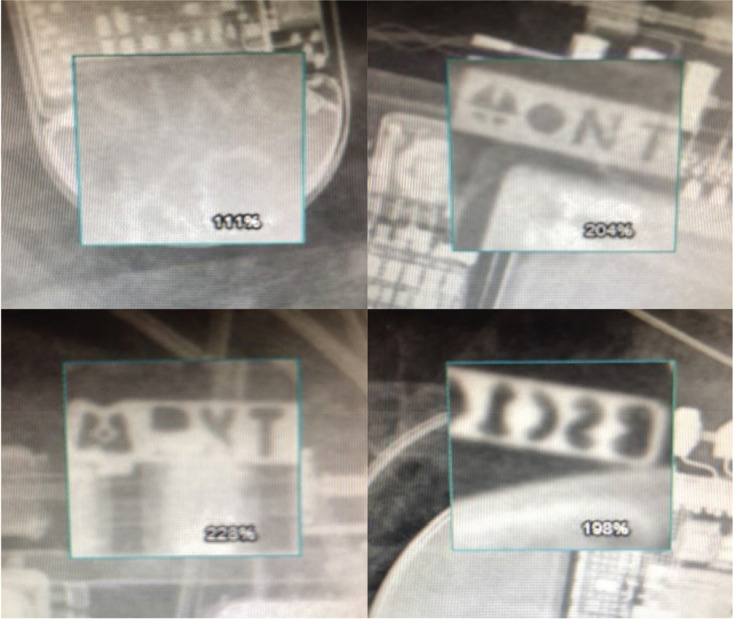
The manufacturer-specific X-ray logos from left to right in clockwise rotation from top left: Abbott, Biotronik, Boston Scientific, and Medtronic.
